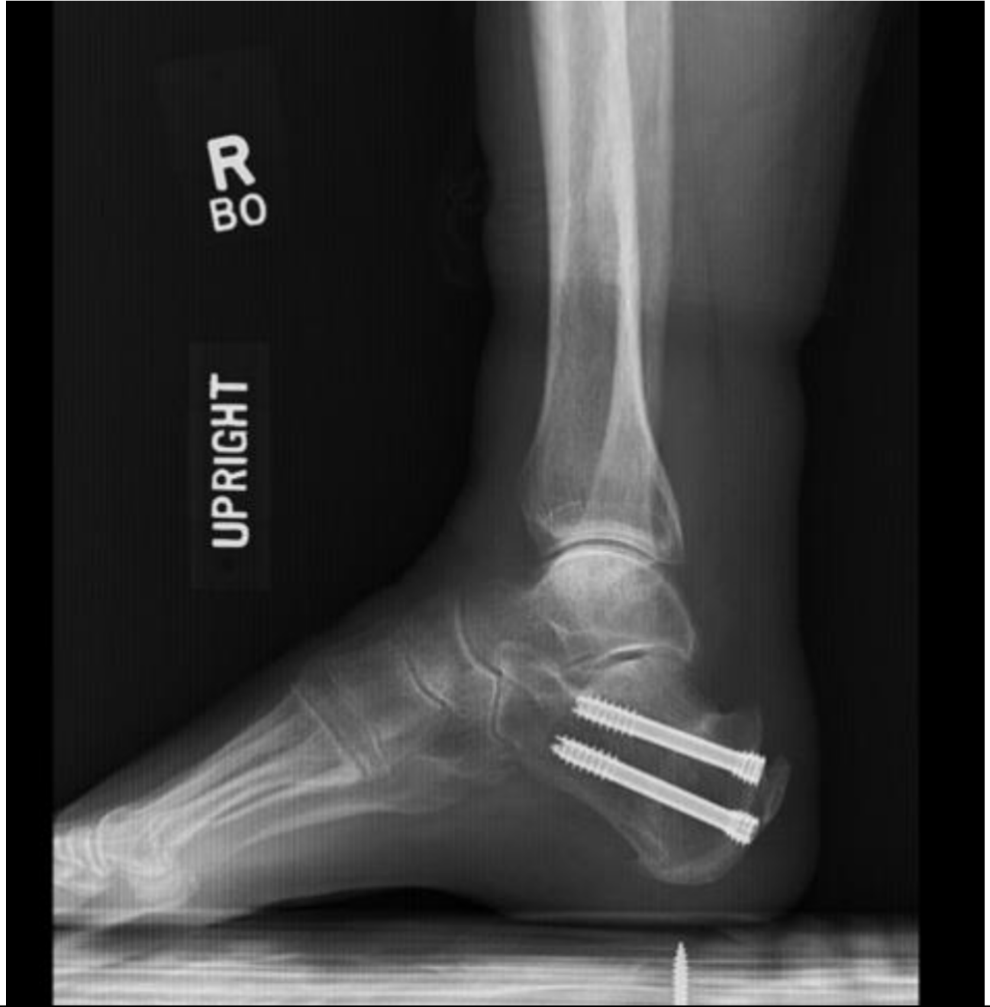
Postoperative lateral radiograph demonstrating two posterior screws and correction of Haglund’s deformity
